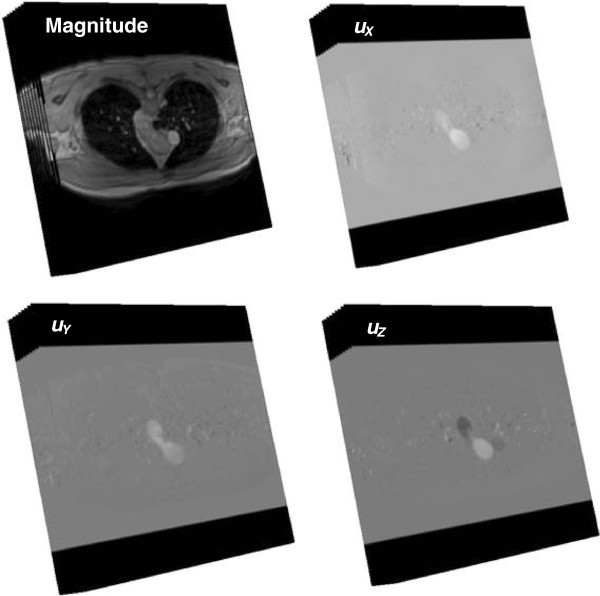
The stack of PC MR images, magnitude images and three directional phase images- AP (anterior to posterior), RL (right to left), and FH (foot to head)- containing the velocity information, *u*_*x*_(t), *u*_*y*_(t), *u*_*z*_(t) , respectively, for the PAs. 24 and 40 phases during the cardiac cycle were recorded per each slice for the control and patient subjects, respectively.
